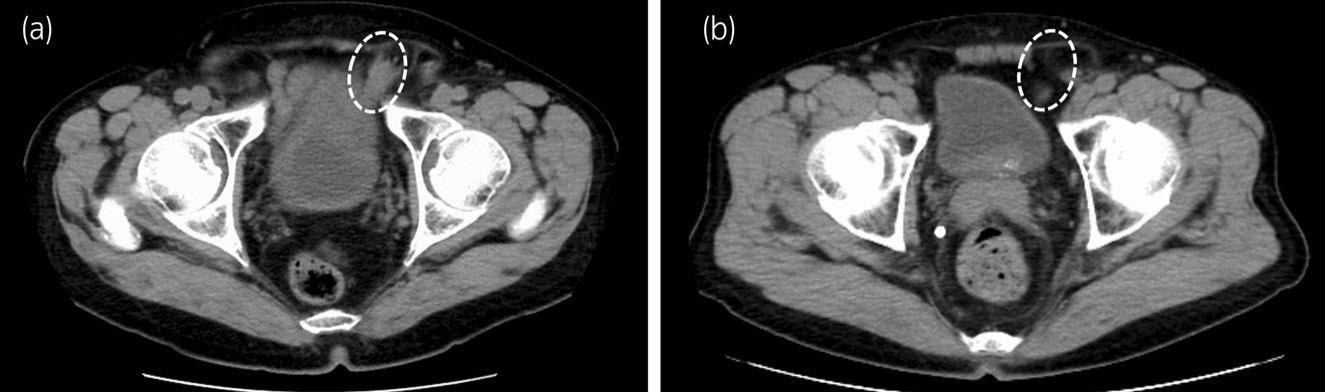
Preoperative CT confirmed left direct inguinal hernias (broken white circles) in both Case 1 (a) and Case 2 (b).
